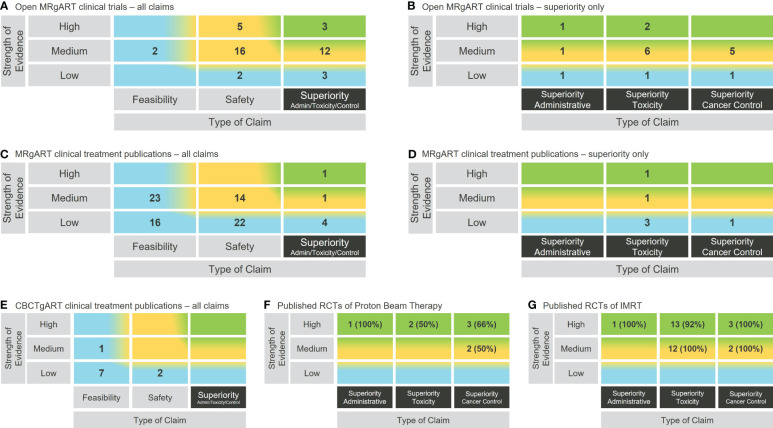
Use of the rtTEM to visualize the clinical evidence status for example RT technologies. (A) Open clinical trials analysis for online and real-time MRgART. (B) Zoomed in view on the open online and real-time MRgART clinical trials superiority claims. (C) Clinical treatment publications analysis for online and real-time MRgART. (D) Zoomed in view on the online and real-time MRgART clinical treatment publications superiority claims. (E) Clinical treatment publication analysis for online CBCTgART. (F) Zoomed in superiority claim analysis of published RCTs on PBT (% of publications that support trial primary endpoint). (G) Zoomed in superiority claim analysis of published RCTs on IMRT (% of publications that support trial primary endpoint).
